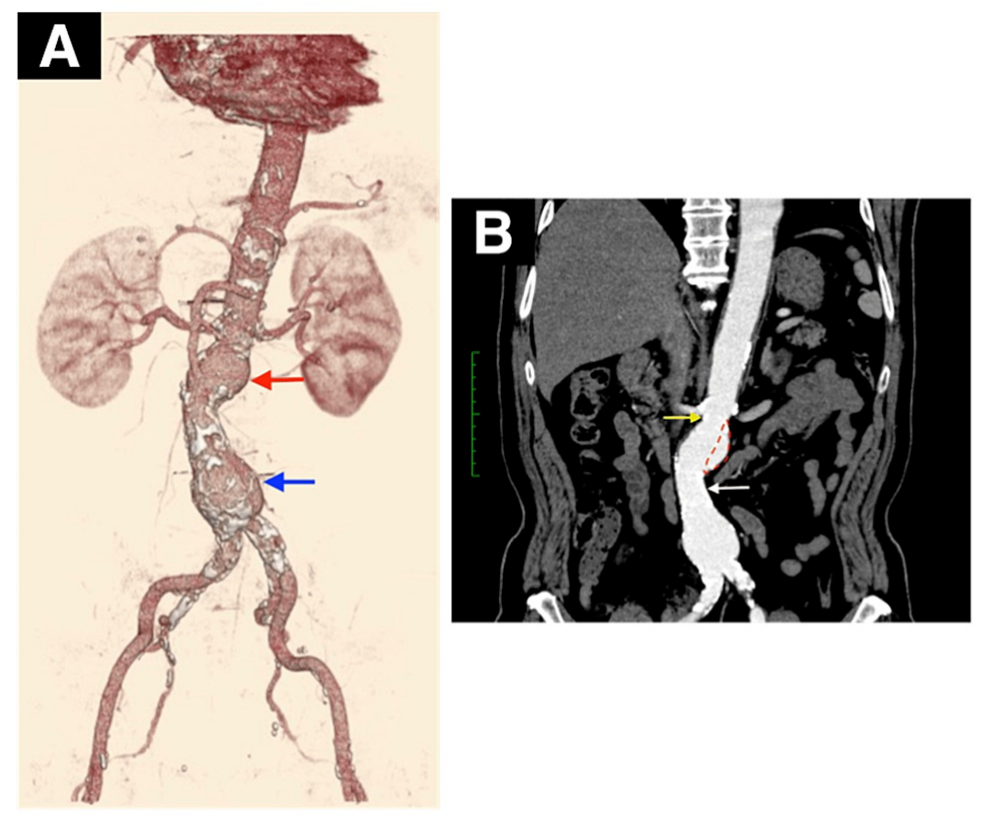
(A) Preoperative three-dimensional reconstruction computed tomography of the abdominal aorta shows severe calcification and multiple abdominal aortic aneurysms.The first aneurysm was 40 mm in diameter (red arrow) and the second aneurysm was 50 mm (blue arrow). (B) The first short neck length was 7 mm (yellow arrow) and second neck length was 30.5 mm (white arrow). The red dotted lines indicate coil embolization areas.
